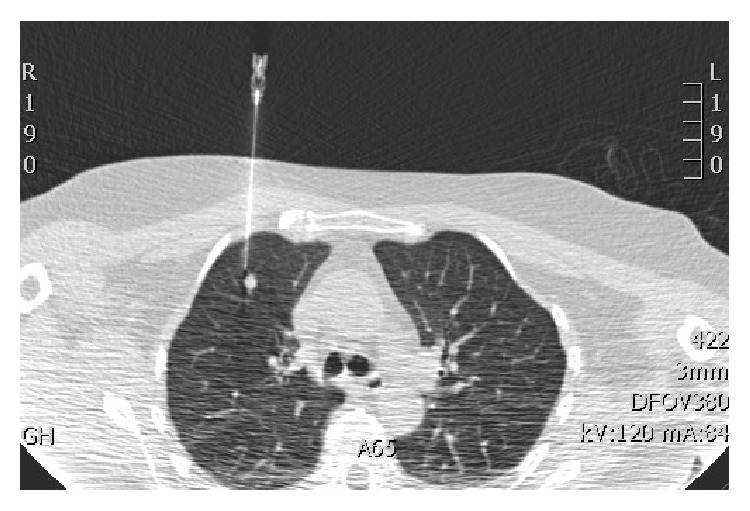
Image capture from CT fluoroscopy: needle inserted under CT fluoroscopic guidance to a position adjacent to the target nodule.
